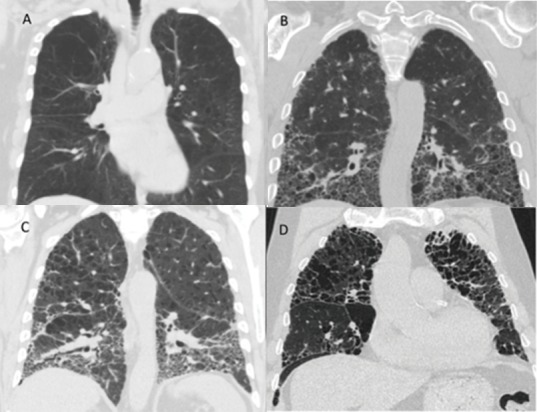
Representative chest computed tomography (CT) images from selected patients from the authors’ institution with emphysema alone (A), pulmonary fibrosis alone (B), upper lobe emphysema with lower lobe pulmonary fibrosis (C), and upper lobe emphysema with thickened fibrotic walls, also referred to as air space enlargement with fibrosis (D).
